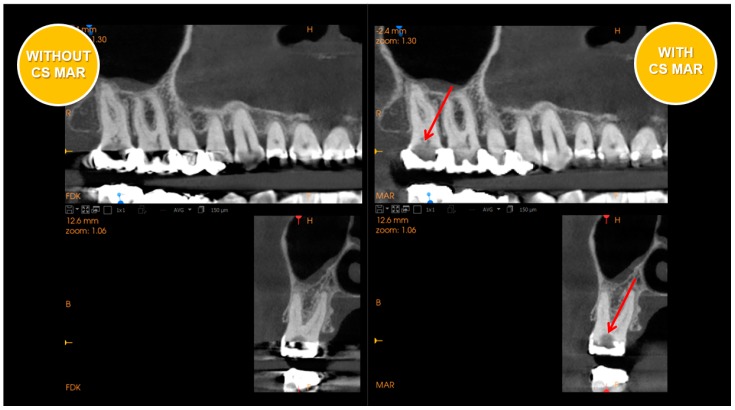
Sagital views of CBCT with and without Metal artifact reduction (MAR) - (Carestream CS9600 MAR).
